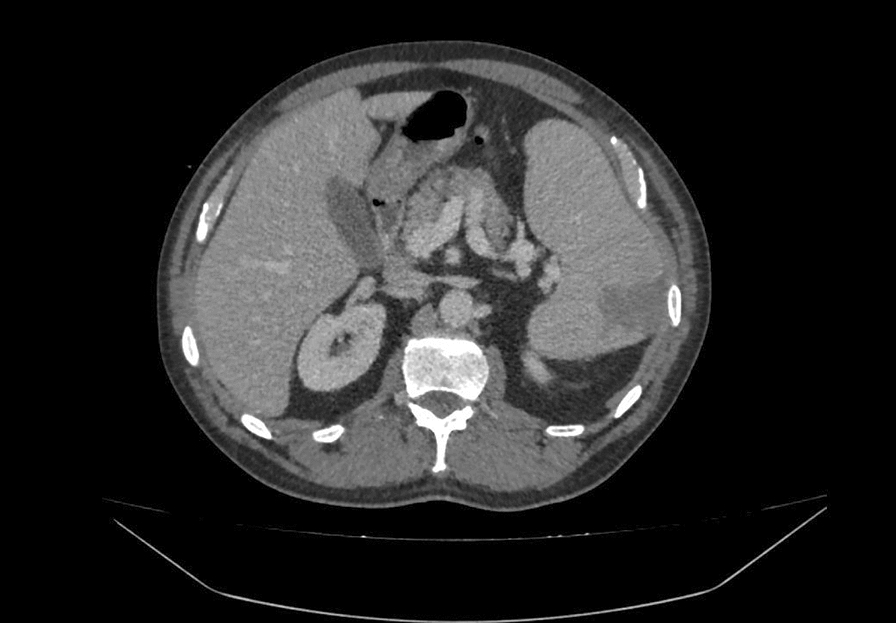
Computed tomography during *Plasmodium ovale* malaria showing splenic infarction
